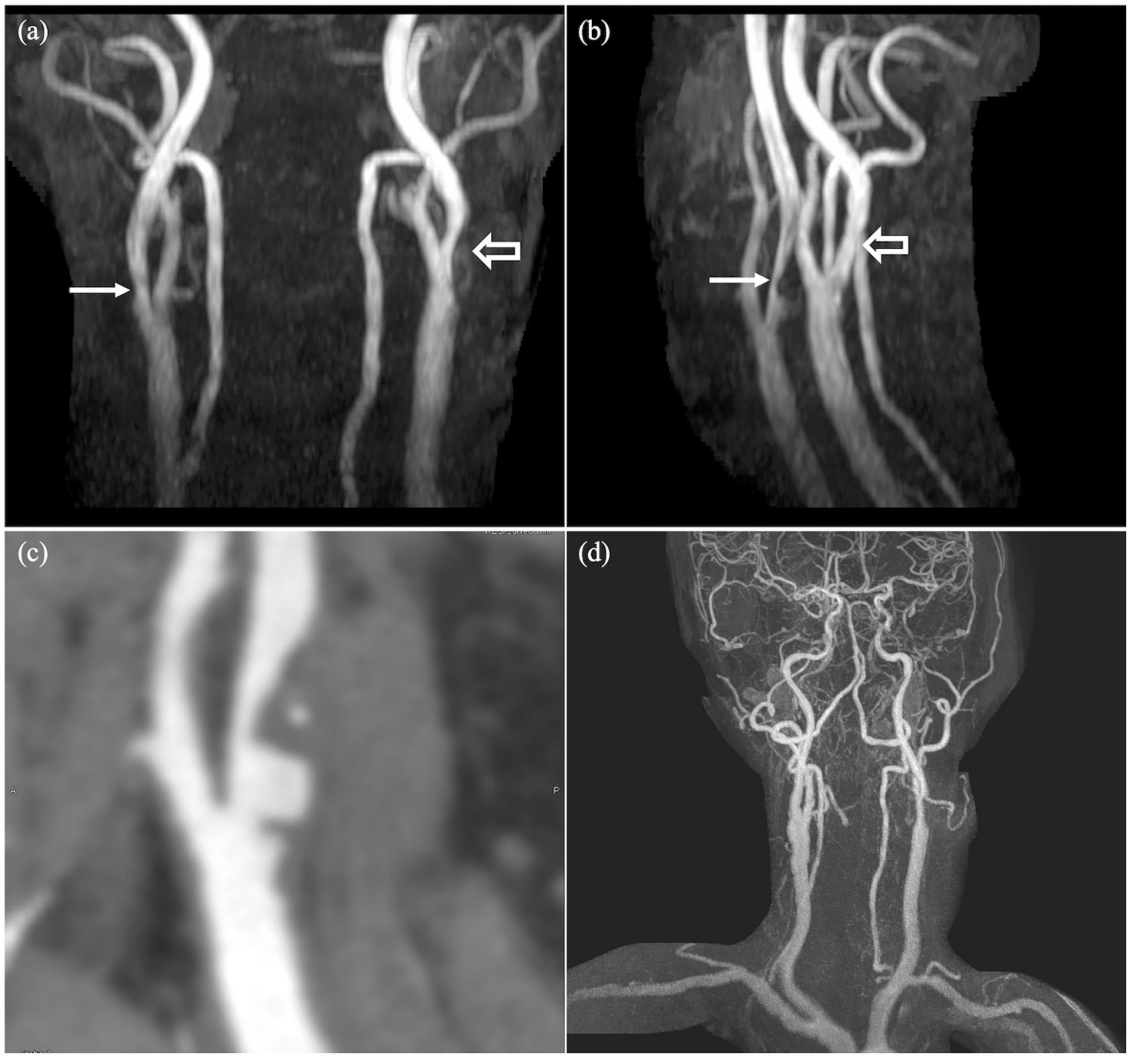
An incidentally detected significant extracranial stenosis in a 71 year-old-male with cognitive impairment. A severe stenosis at right proximal ICA (arrows) and a mild stenosis at left proximal ICA (open arrows) are noted on TOF-MRA (a,b). CT angiography demonstrates non-calcified plaque at right proximal ICA resulting in severe stenosis (c). Contrast-enhanced MR angiography after carotid endarterectomy shows successful dilatation of the right ICA (d).
